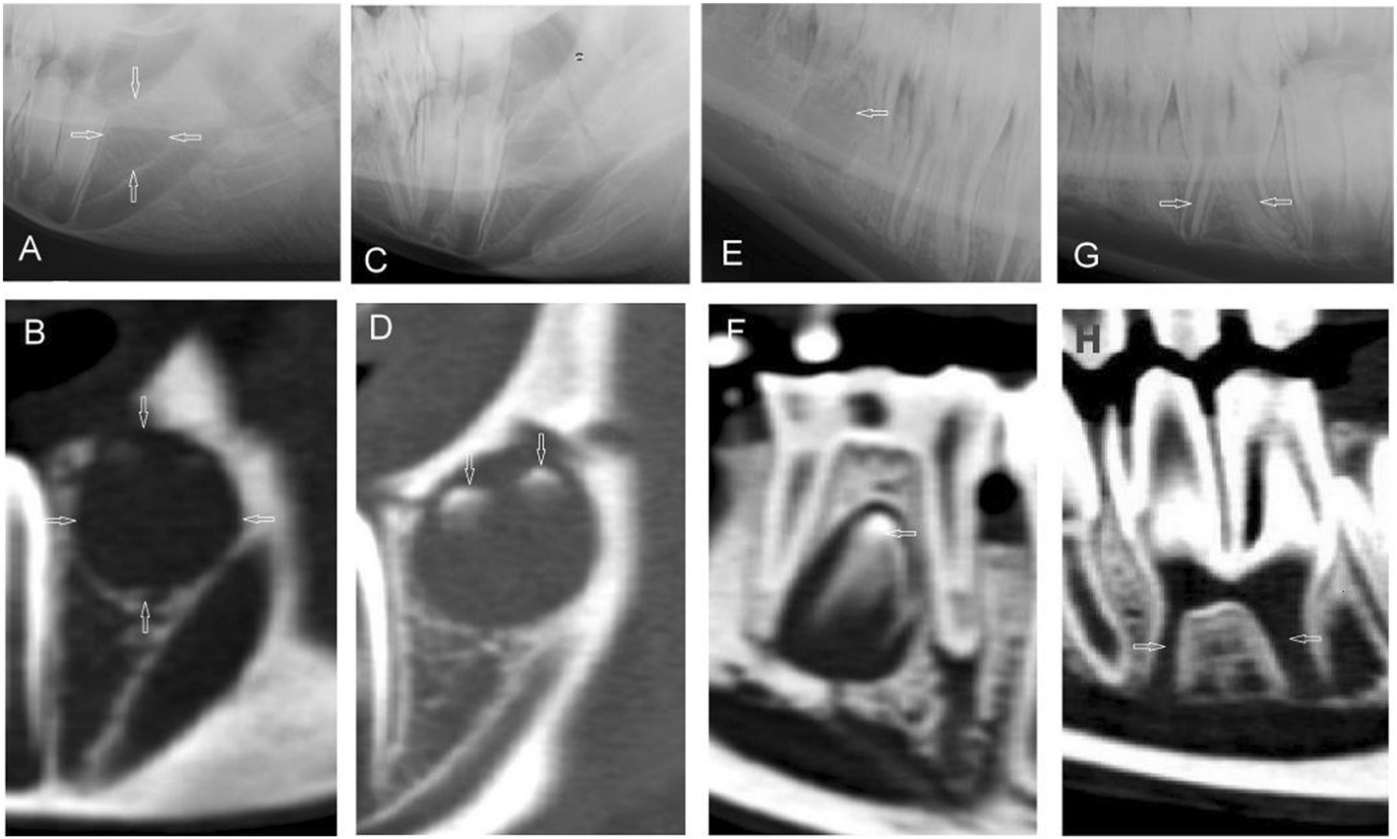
Different stages of the development of the dental sac. Lateral30°ventral - laterodorsal radiographs (upper row) and corresponding sagittal CT-reconstructions (lower row) of the development of the dental sac in different stages. A, B: Early radiolucent dental sac for mandibular 10′s in a 9 month old alpaca. C, D: Coronal mineralization of the mandibular 10 with two enamel columns in a 9 month old llama (not visible in C). E, F: Erupting mandibular permanent 08 in a 30 month old llama, which would cause the deciduous tooth shedding down later. G, H: Wide pulp cavities and a common pulp chamber with open apical foramina in a recently erupted mandibular 08 in a young llama (age 32 months). Described findings are indicated with white arrows.
